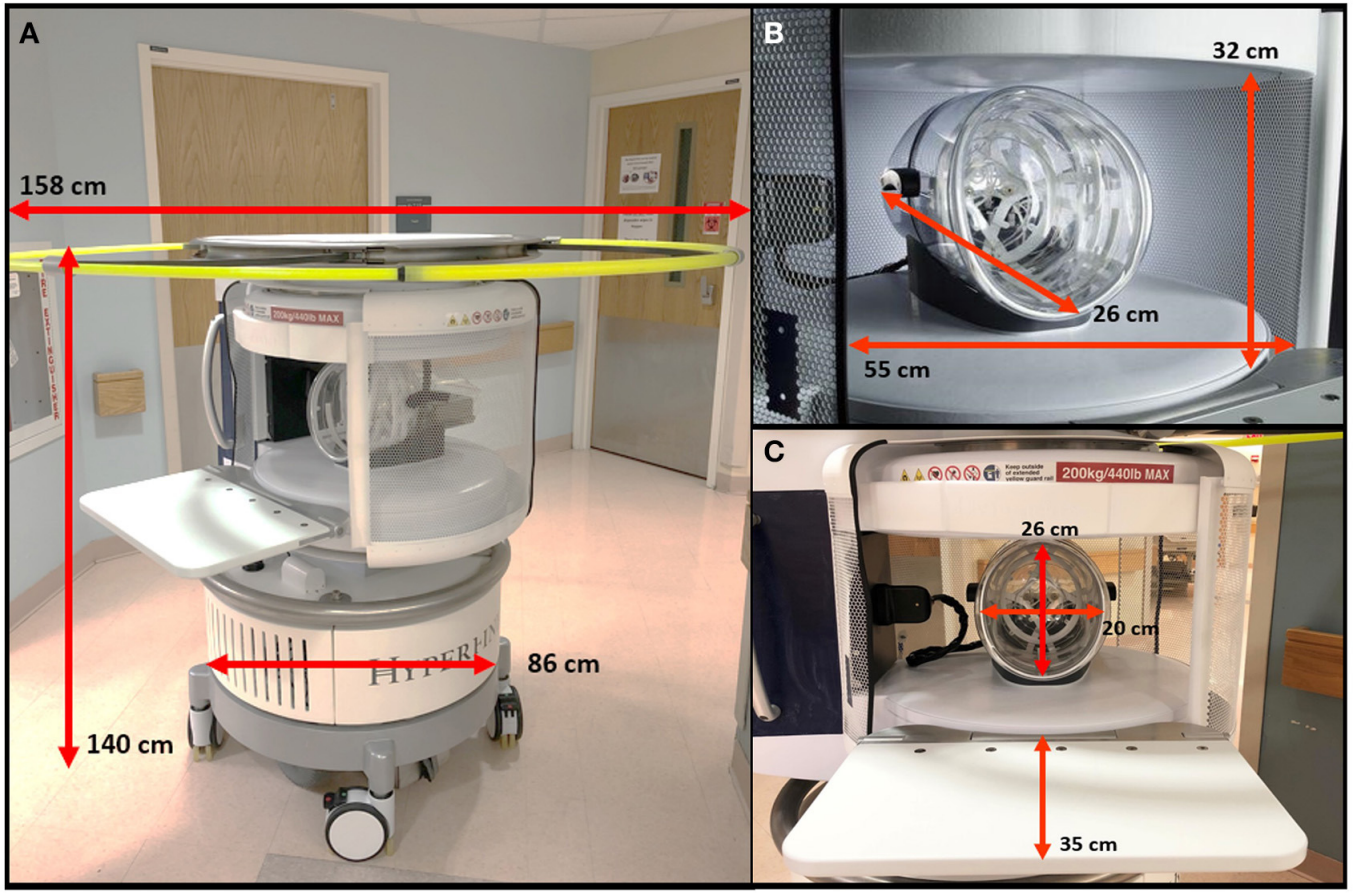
Portable MRI dimensions. (A) The pMRI device has a height of 140 cm and a width of 86 cm. There is an expandable ring Gauss Guard, with a diameter of 158 cm, which demarcates the 5 Gauss (0.5 mT) boundary. (B) The vertical space between the two magnets is 32 cm, within which there is a head coil of 26 cm length. The horizontal width between the RF shield, which is closed during scan acquisition, is 55 cm. (C) During a scan, the patient's head is positioned within the head coil (26 × 20 cm), and the scanner bridge (35 cm) is extended to adjoin with the patient's hospital bed.
